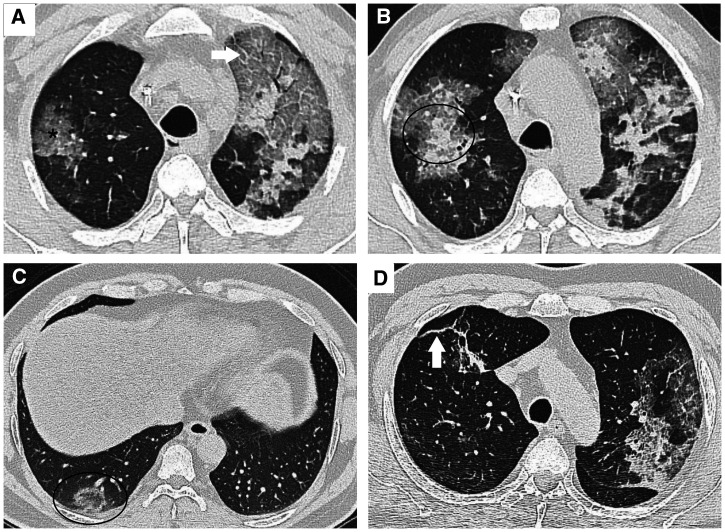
Axial sections of computed tomogram of chest of COVID-19 patients. (A) an area of ground-glassing (asterisk) and prominent interlobular septae suggestive of “crazy-paving” pattern (arrow). (B) An area of consolidation surrounded by ground-glassing, the halo sign (encircled area). (C) An area of ground-glassing surrounded by a rim of consolidation, the reverse halo sign. (D) Fibrotic strands (arrow).
